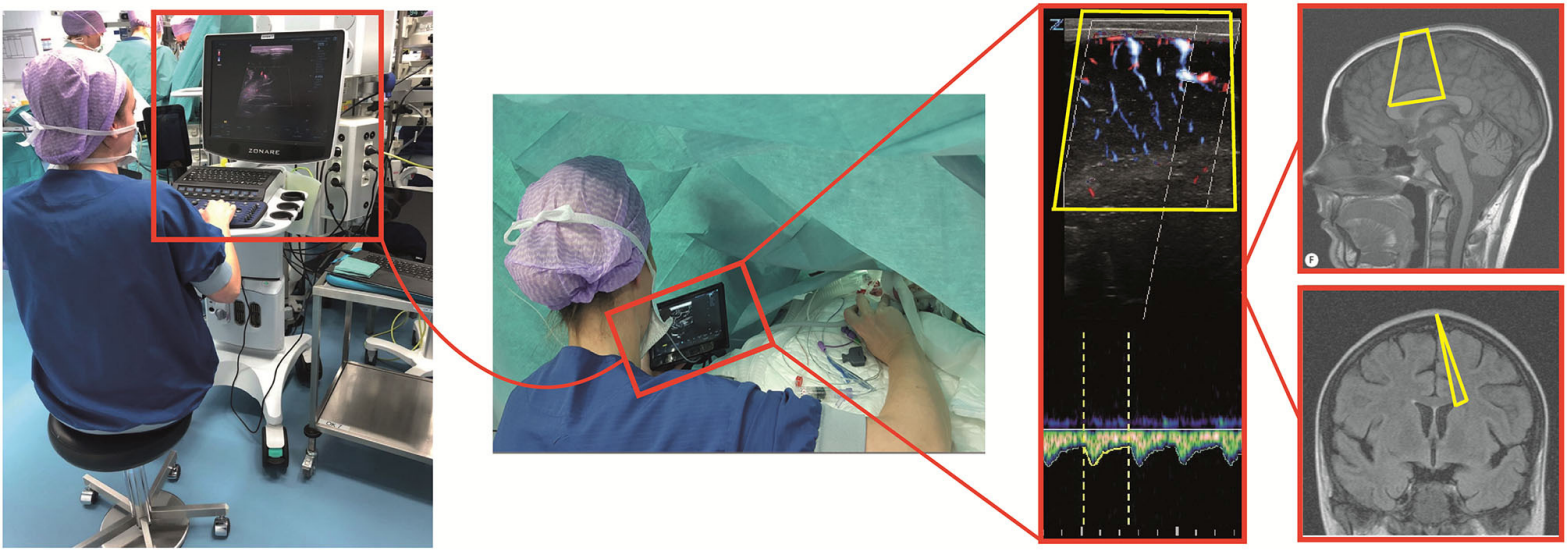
Ultrasound measurement setup. (Left) One researcher controlled the ultrasound machine outside the workspace of the surgeon and anaesthesiologist. (Middle) One researcher held the probe and performed the ultrasound scan with the feedback by an extended screen. (Right) The cerebral vasculature in the ultrasound plane was visualized with power Doppler. The spectral Doppler gate was placed in a vertically oriented part of this pial artery.
